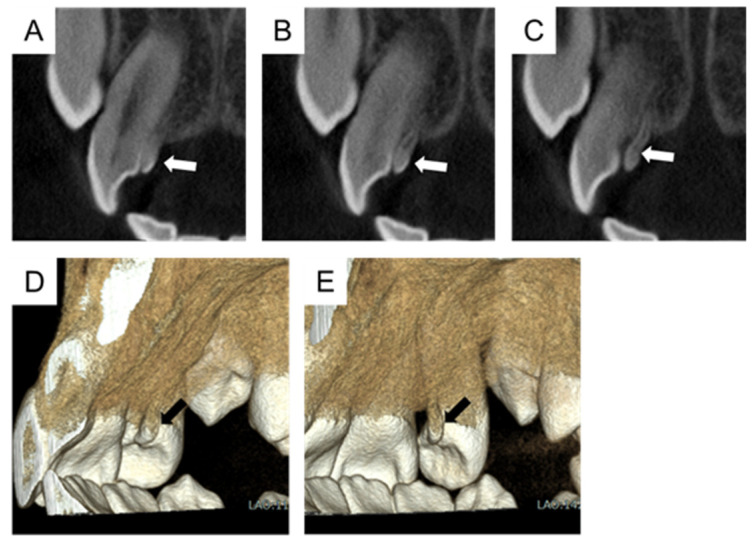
Cone-beam computed tomography imaging of the sagittal section and three-dimensional construction images at the age of 11 years and 6 months. (A–C) The sagittal section. (D,E) Three-dimensional construction images. Arrows indicate the fused tooth with the supernumerary tooth.
